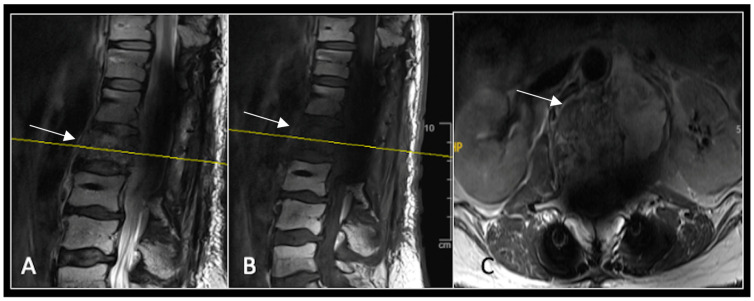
A 12-month postoperative surveillance MRI shows no evidence of residual tumour (arrow) disease progression. The severity of artefact is reduced. The yellow line on the sagittal images denotes the level of the axial image. (A) T2W sagittal; (B) T1W sagittal; (C) T2W axial.
